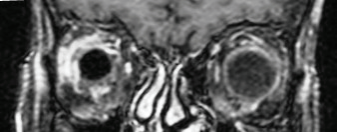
RE-MRI aspect after PMMA ball-dacron surgery
